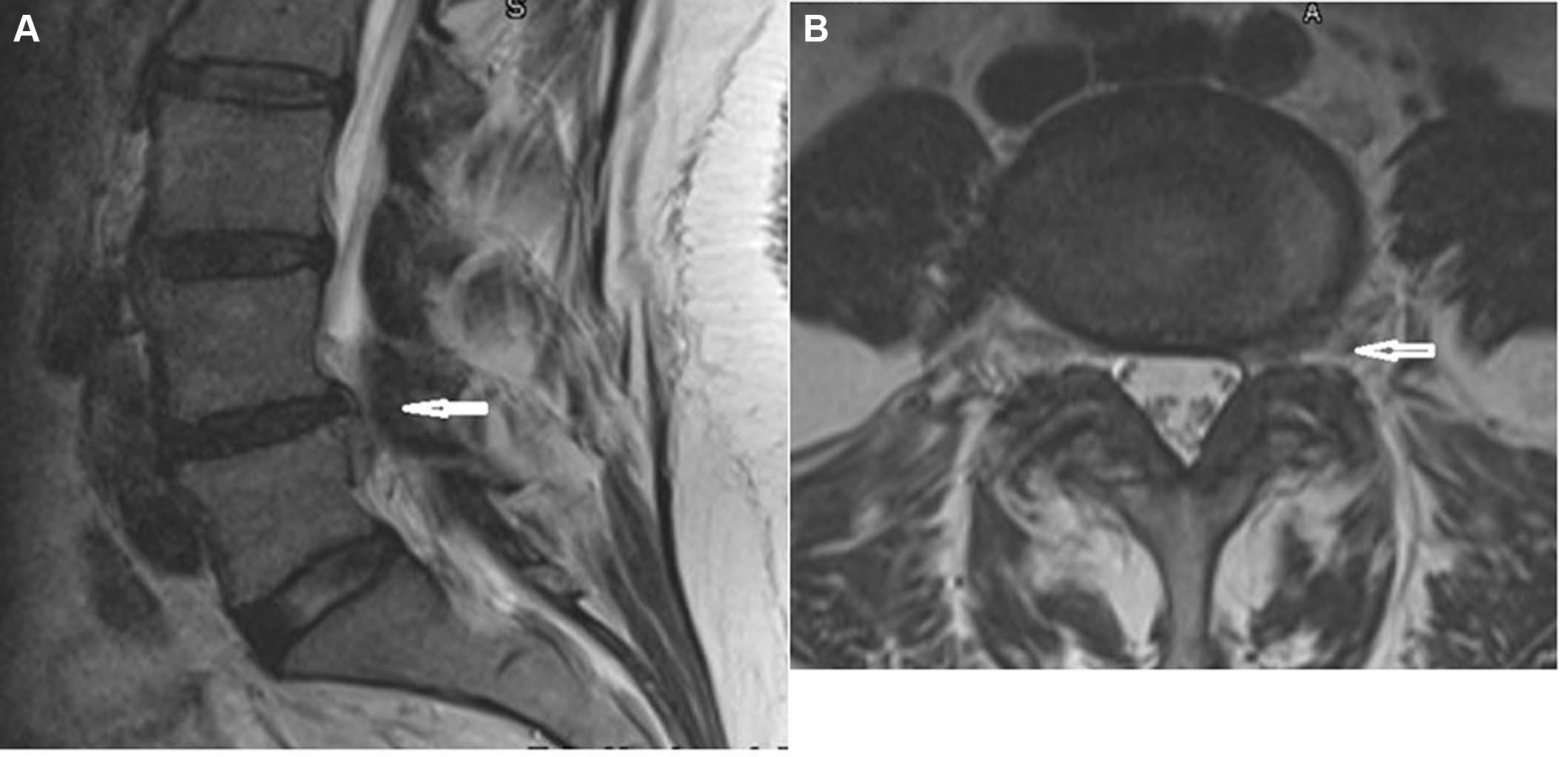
MRI of lumbar spine. (A) Sagittal view. (B) Axial view L4–L5.
